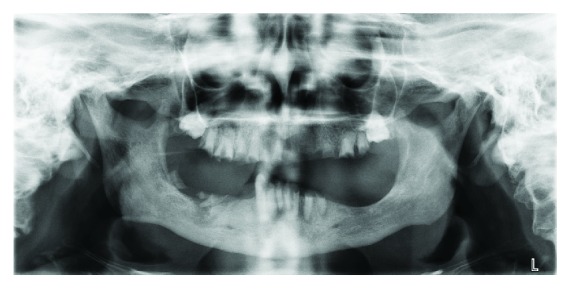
Orthopantomogram (OPG) July 2018 showing grossly carious retained roots and associated periapical pathology. Unerupted maxillary right and left third molars are also evident (18, 28).
